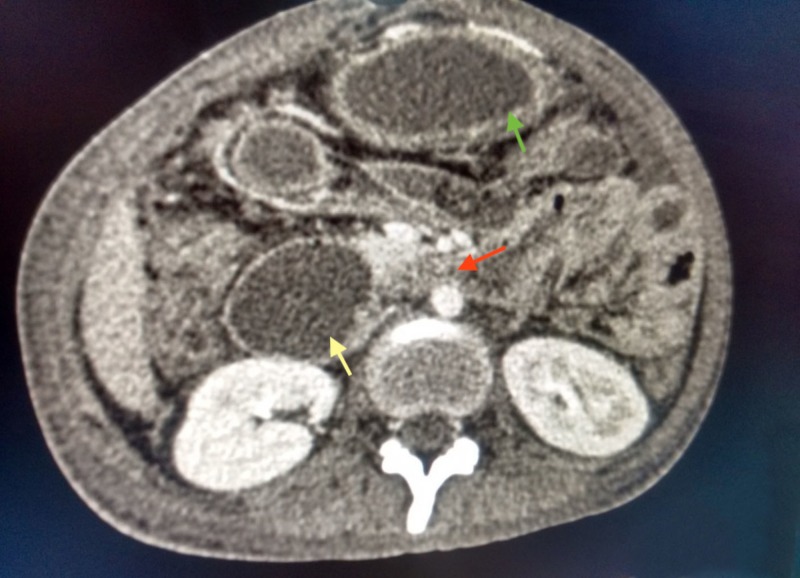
Axial view of contrast enhanced computed tomography of abdomen showing compression of third part of the duodenum (red arrow) with gastric dilation (green arrow) and proximal duodenal dilation (yellow arrow).
